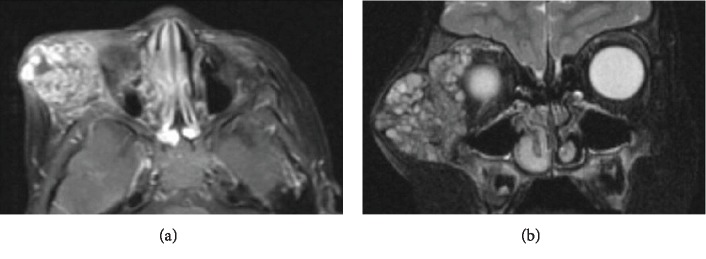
(a) Axial T1-weighted postcontrast fat-saturated magnetic resonance (MR) image shows avid heterogenous enhancement of the soft tissue and intraosseous components. (b) Coronal T2-weighted fat-saturated noncontrast MR image shows the mass with multiple septations/cystic components.
